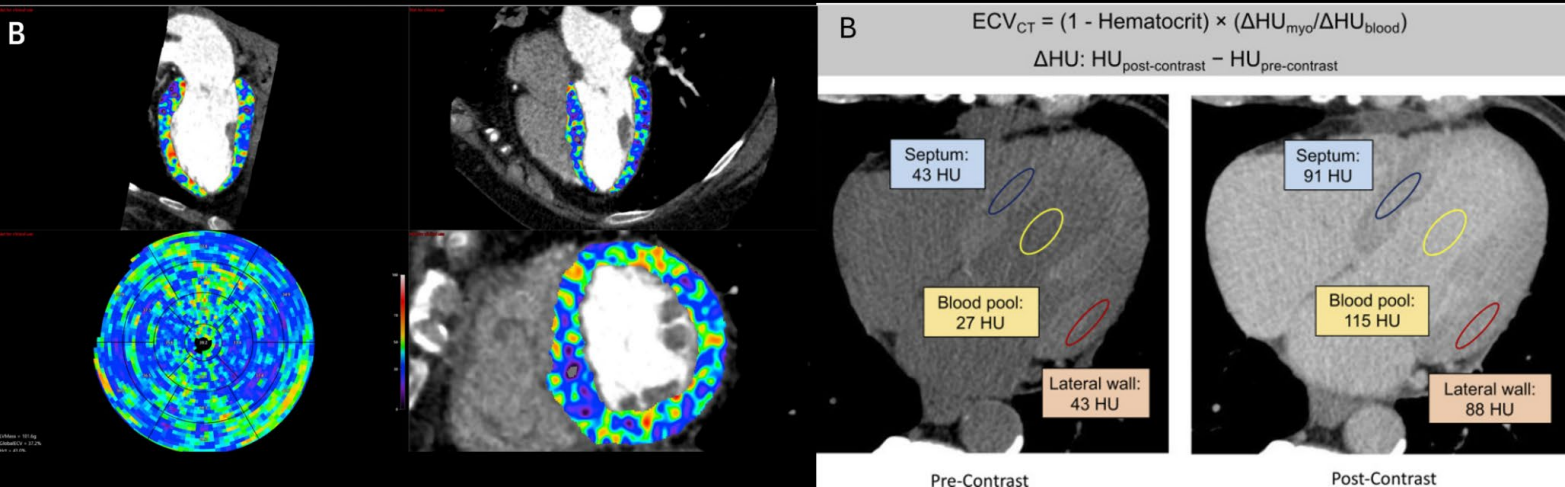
Global versus Local Measurement of Extracellular Volume using Computed Tomography. Images showing global versus local measurement of extracellular volume on CT. In (A) specialized software calculates CT-ECV values for each segment of the myocardium according to a 17-segment model which provides an understanding of regional variation in fibrosis. In (B) ROIs from the septum or lateral wall can be used to calculate local CT-ECV values. This does not require specialized software. The septum is often chosen to avoid beam hardening artifact from the spine. The formula used to calculate CT-ECV is demonstrated at the top of panel B
